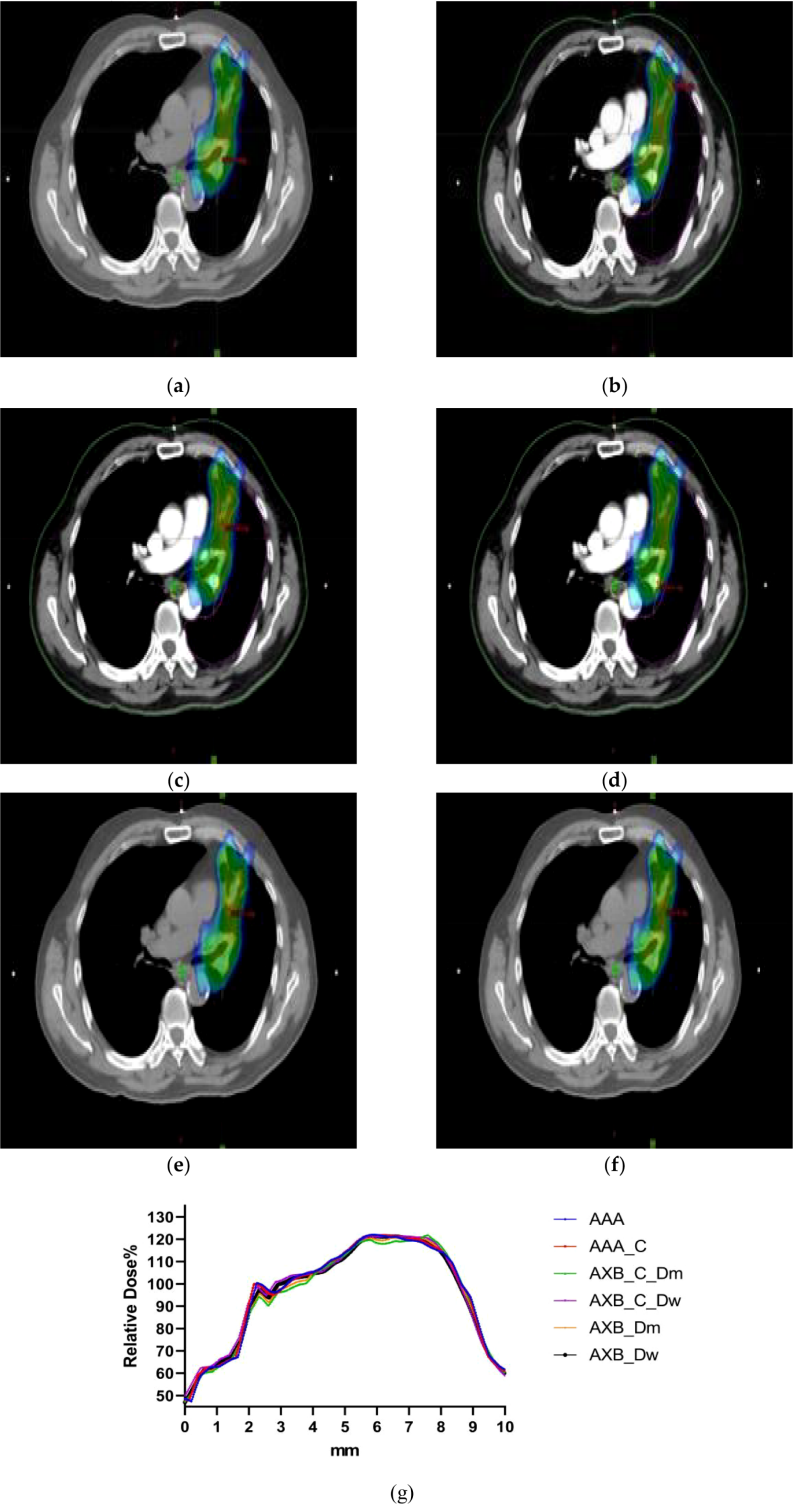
(a–f)The dose distribution maps of the AAA algorithm and AXB algorithm in the Dw and Dm dose reporting modes under contrast-enhanced CT and non-contrast enhanced CT conditions. (g) Characteristic dose curves around the tumor of a patient under different calculation conditions.
